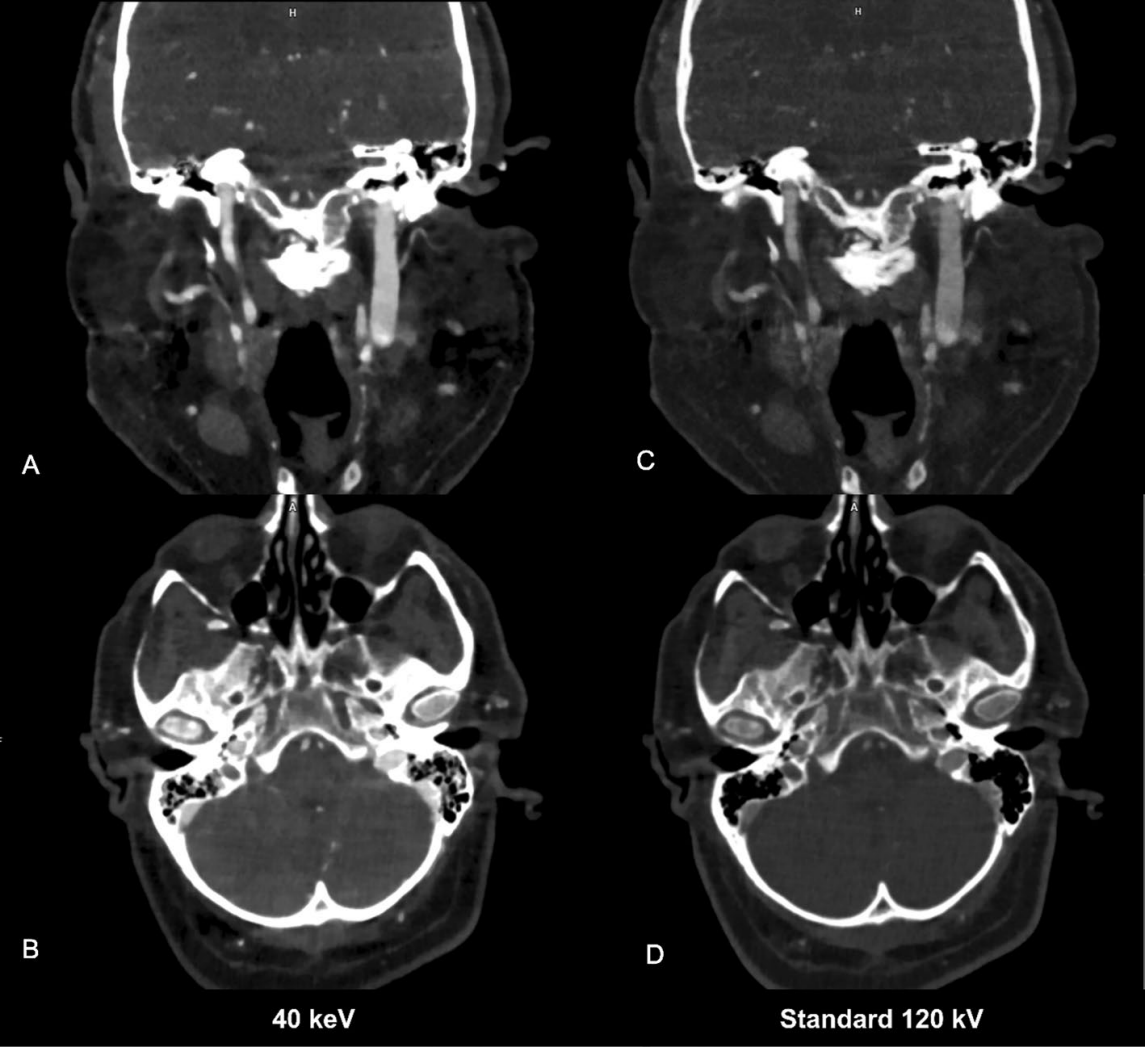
A 78-year-old female with aortic valve stenosis and comorbidities including diabetes mellitus type 2, and chronic kidney disease, undergoing PCCT angiography pre-TAVI, using 80 ml of 350 mgI/mL Xenetix at an infusion rate of 5.0 ml/second (Xenetix 350 mgI/ml, Guerbet, Villepinte, France). PCCT angiography reconstructions in paracoronal and axial plane (A–B) at low-VMI energy levels 40 keV show less amount of hypoattenuating artifacts and a regular opacification of right ICA, clearly indicating a regular perfusion, compared to paracoronal and axial reconstructions (C–D) in standard 120 kV. *ICA* Internal Carotid Artery; *PCCT* Photon-counting CT; *TAVI* Transcatheter Aortic Valve Implantation
